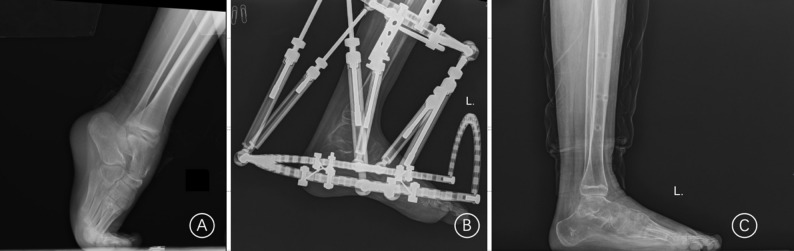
Typical case 5, rigid equinus deformity related to Fibro-Adipose Vascular Anomaly. A preoperative lateral X-ray films. B postoperative lateral X-ray films. C lateral X-ray films after fixator removal
